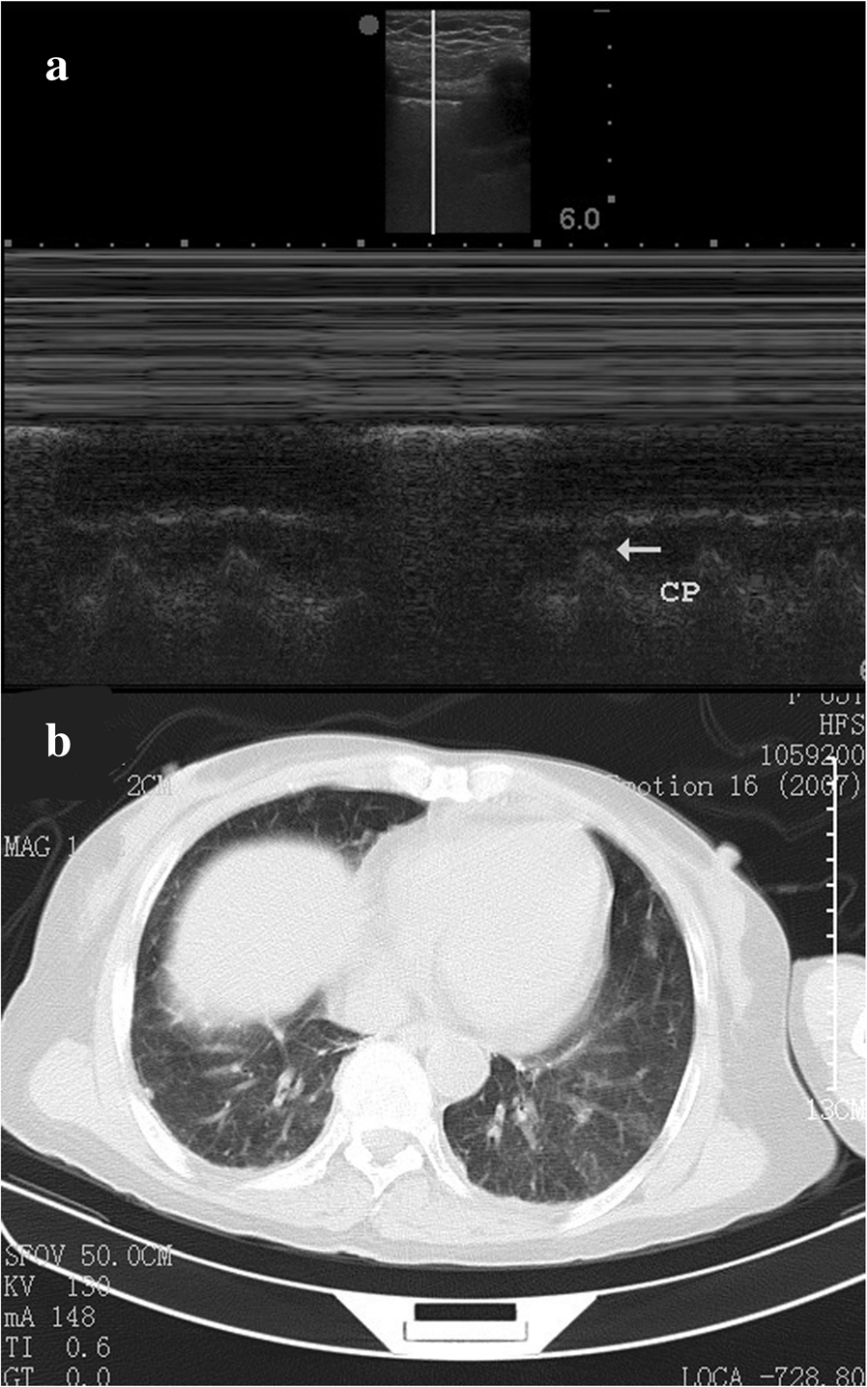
Real-time mode ultrasonography and computed tomography. (a) Real-time mode showed intermittent eradication of cardiac pulse (CP) by the moving lung with respiration cycle. (b) Subsequent computed tomography ruled out pneumothorax.
